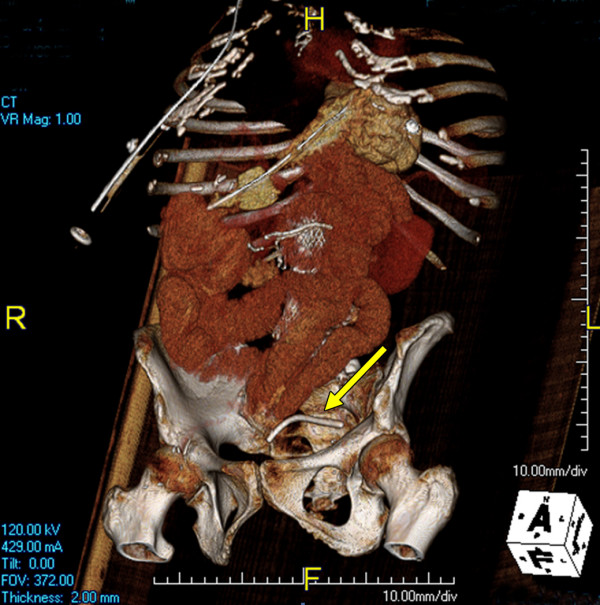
CT Scan of the common bile duct stent. 3-Dimensional reconstruction of CT data demonstrating the migrated biliary stent to be extraluminal in the left lower quadrant.
